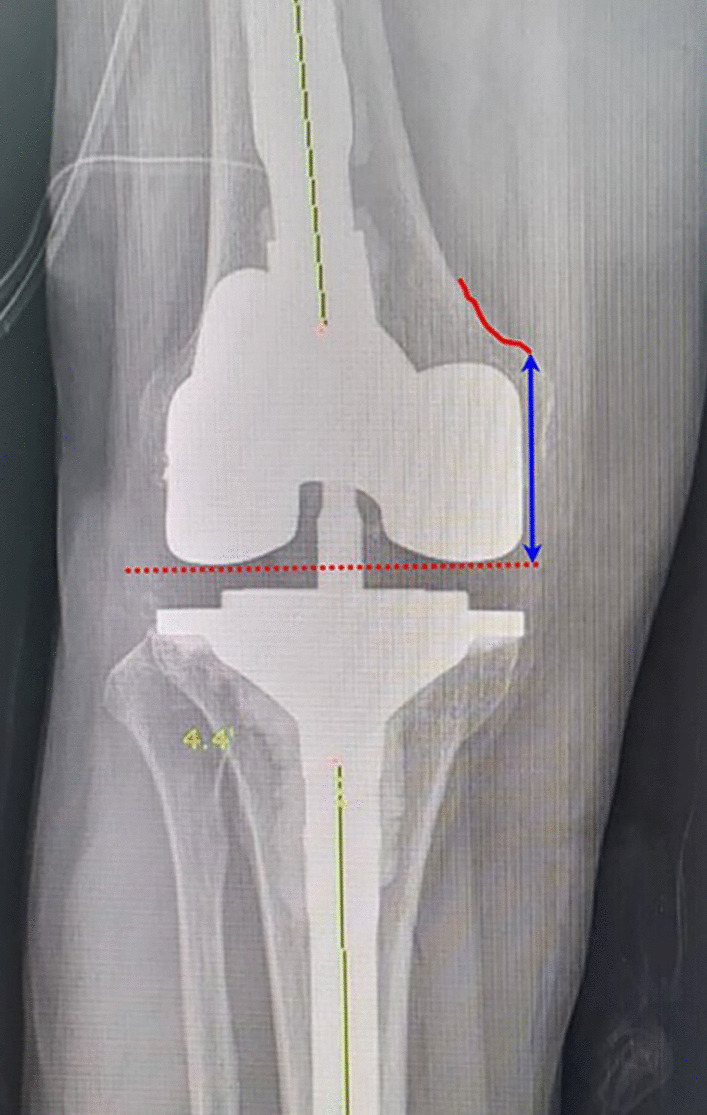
Femoral joint line position was defined as the distance from the adductor tubercle to the joint line in an anteroposterior radiograph (blue-colored arrow)
